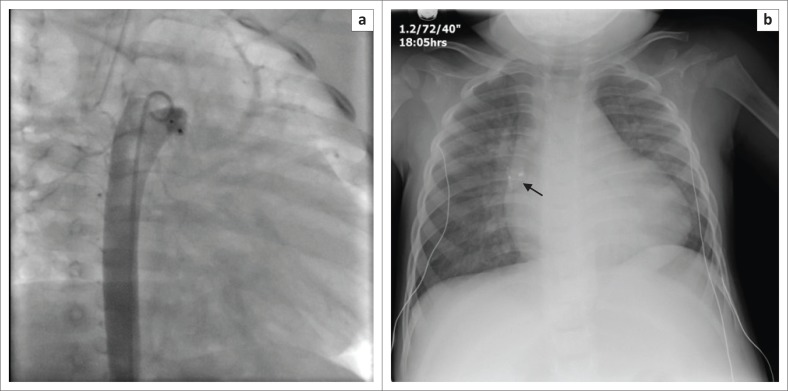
A migrated amplatzer duct occluder placed for patent ductus arteriosus. Aortogram in the oblique anterior–posterior view (a) shows transcatheter deployment of the amplatzer duct occluder in the lumen of the aorta. Post-procedural chest radiograph (b) shows migration of the device (arrow).
